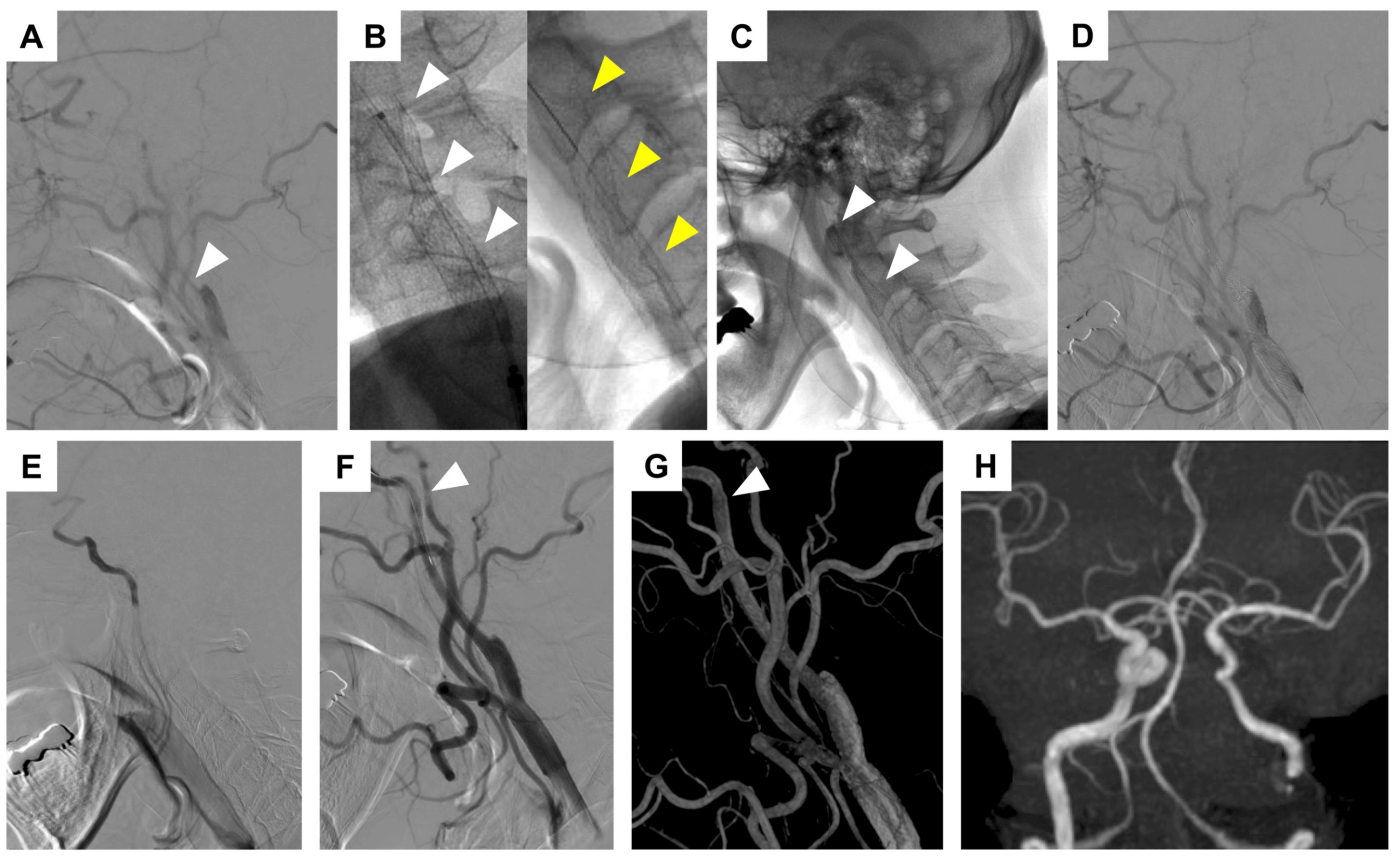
Intraoperative angiograms and postoperative imaging findings of second CAS A: Preoperative left CCA angiogram. The arrowhead indicates severely slow flow in the left ICA; B: Radiographic images immediately after stent placement and prior to the second CAS. The white arrowhead indicates the initial stent, and the yellow arrowhead indicates the stent prior to the second CAS; C: Radiographic image after stent placement. The arrowheads indicate additional closed-cell stents; D: CCA angiogram after the placement of the additional stent; E: Microcatheter angiography in the normal left ICA distal to the dissection site; F-G: Final angiogram and 3D-RA image. The arrowhead indicates the most distal part of the second closed-cell stent; H: Head MRA performed the day after the second CAS, demonstrating normalization of ICA visualization. CAS: carotid artery stenting; CCA: common carotid artery; ICA: internal carotid artery; MRA: magnetic resonance angiography; 3D-RA: three-dimensional rotational angiography
